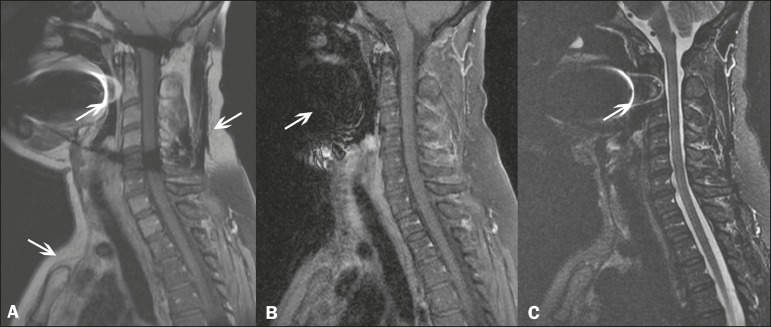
Sagittal images of the cervical spine of a 52-year-old female patient with various pulse sequences: contrast-enhanced T1-weighted FSE with fat suppression (A), contrast-enhanced T1-weighted IDEAL (B) and STIR (C). The IDEAL sequence (B) shows a clear reduction of the magnetic susceptibility artifact, especially for visualization of the second cervical vertebra.
